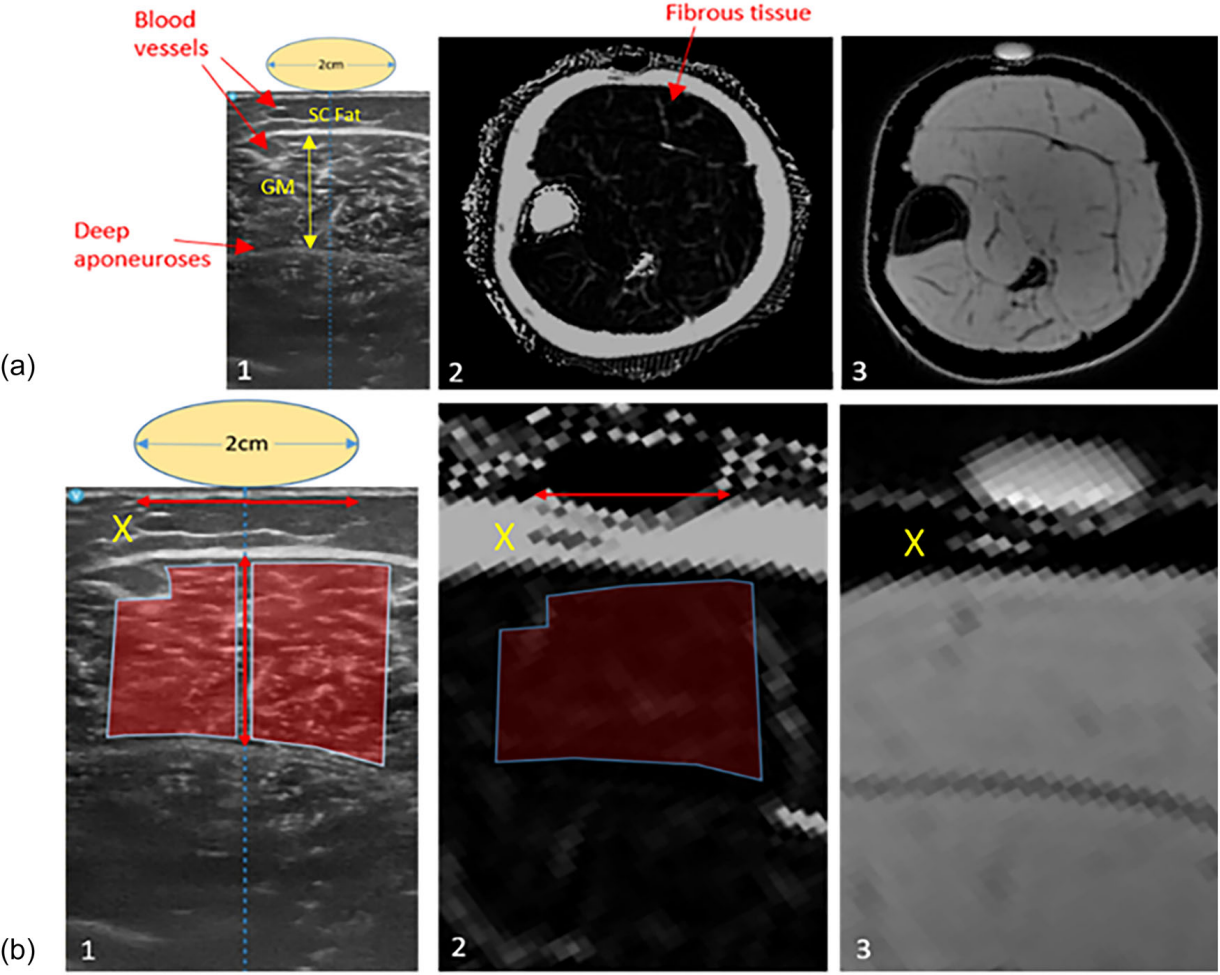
(a) Gastrocnemius medialis (GM) ultrasound anatomical identifiers, thickness and subcutaneous (SC) fat layer (1), MRI fat fraction map (2), and MRI water fraction map (3). (b) The respective magnified locations for measurements of ultrasound echointensity (1), fat fraction percentage (2) and water image (3) for reference. X, matching anatomical landmark.
